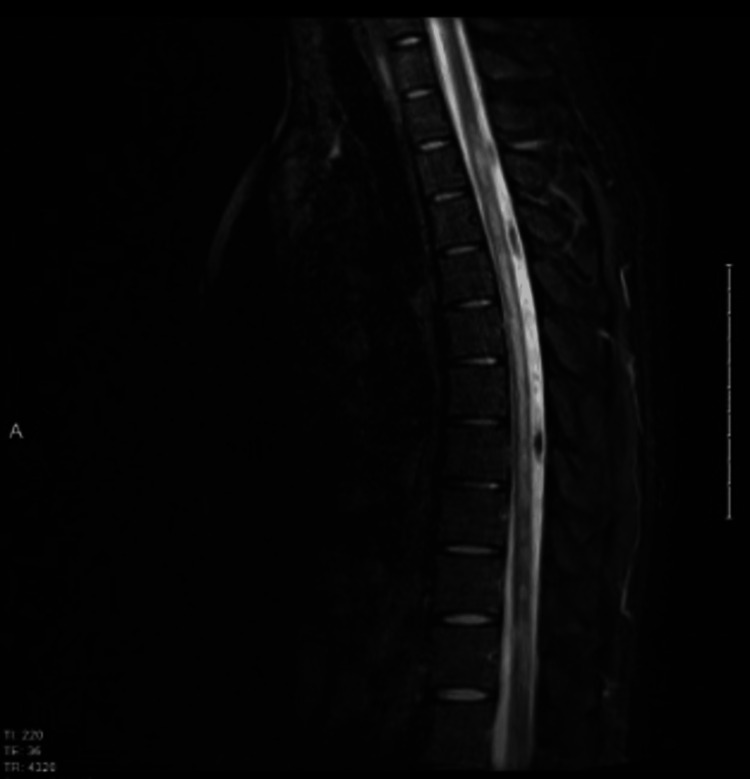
MRI of the thoracic spine T2 with contrast. It shows hyperintense signal intensity with enhancement post gadolinium
